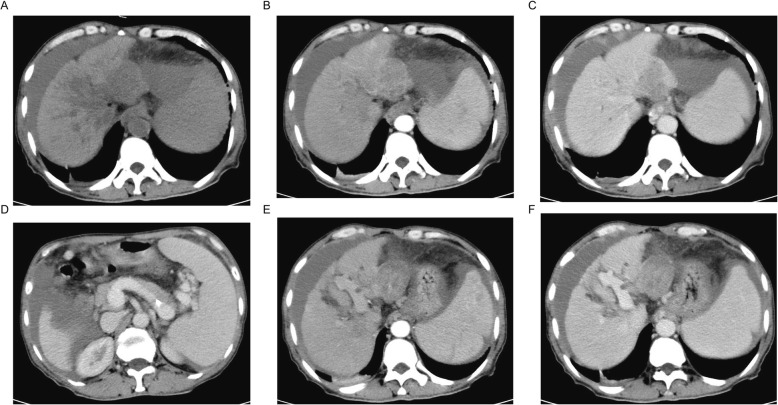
CT of the abdomen showing the segment 3 liver lesions at diagnosis. a The characteristics of liver mass in plain scan; b Liver mass in arterial phase; c Liver mass in portal phase; d Multiple collateral circulations; e Partial thrombosis of the left portal vein in arterial phase; f Partial thrombosis of the left portal vein in portal phase
